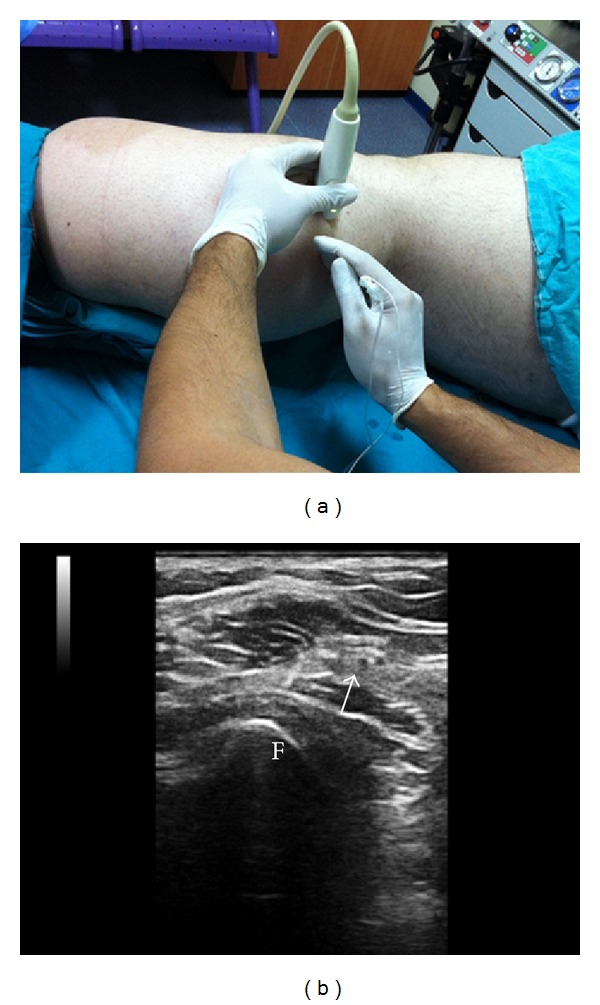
(a) Ultrasound probe placement for common peroneal nerve block at the head of the fibula. (b) Sonogram of the common peroneal nerve at the head of the fibula (arrow). F: fibula.
